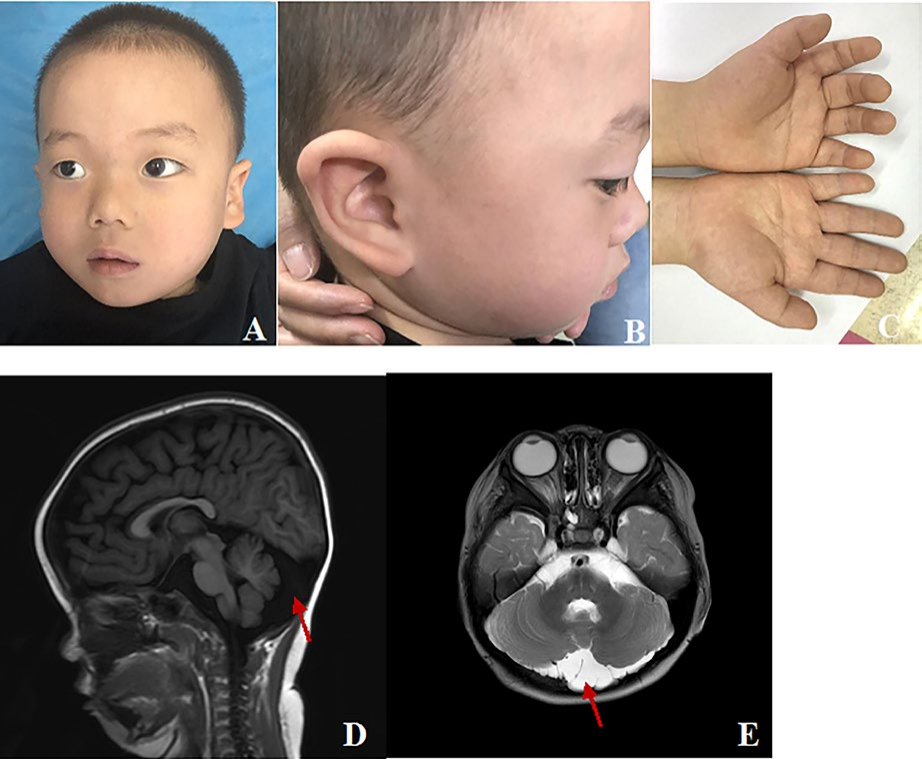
Clinical features of the case 2. (a, b) He had dysmorphic features including a broad forehead, hypertelorism, upslanting palpebral fissures, hypoplastic columella and ala nasi, flat nasal bridge, long philtrum, upturned earlobes, high‐arched palate, micrognathia. (c) He had brachydactyly and simian crease in both palms. (d, e) Brain magnetic resonance imaging (MRI) showed an enlarged cerebellomedullary cistern and arachnoidal cyst
